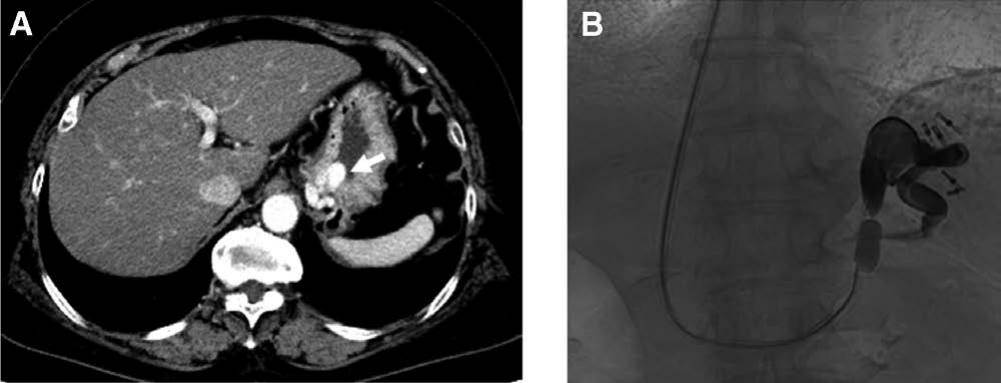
(A) Axial CT images acquired at portal venous phase demonstrating large fundal GV (arrow). (B) Fluoroscopy, showing partial thrombosis of the gastric varices. The gastric varices and gastrorenal shunt are fully opacified by the sclerosant with contrast medium during TJO. CT = computed tomography, GV = gastric varices, TJO = transjugular retrograde obliteration of gastric varices.
